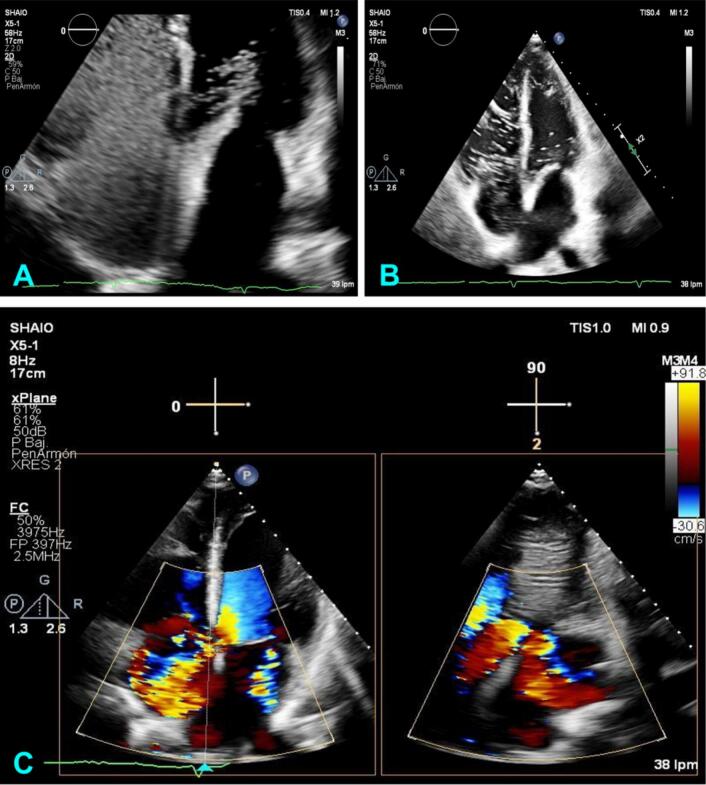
“Transthoracic echocardiogram”. A-B. Study contrasted using shaken saline solution with continuity solution of 7 mm with left ventricle to right atrium doppler flow, negative contrast in the right atrium and bubble movement to the left ventricle during diastole.
